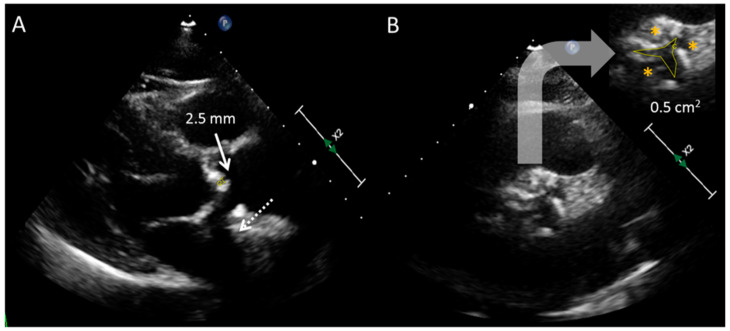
Morphological evaluation of the aortic valve by TTE. (A) Parasternal long-axis view. Extensive calcification of the aortic valve leaflets is noted (dashed arrow: acoustic shadow generated by the calcium). Maximal systolic separation between cusps reaches only 2.5 mm, a finding supporting the existence of a significant stenosis (classical cut off for severe AS: <7–8 mm). (B) Parasternal short-axis view. Three leaflets (asterisks) are identified; at the systole, the aortic valve orifice is significantly reduced (area by direct tracing or “planimetry” is 0.5 cm^2^).
